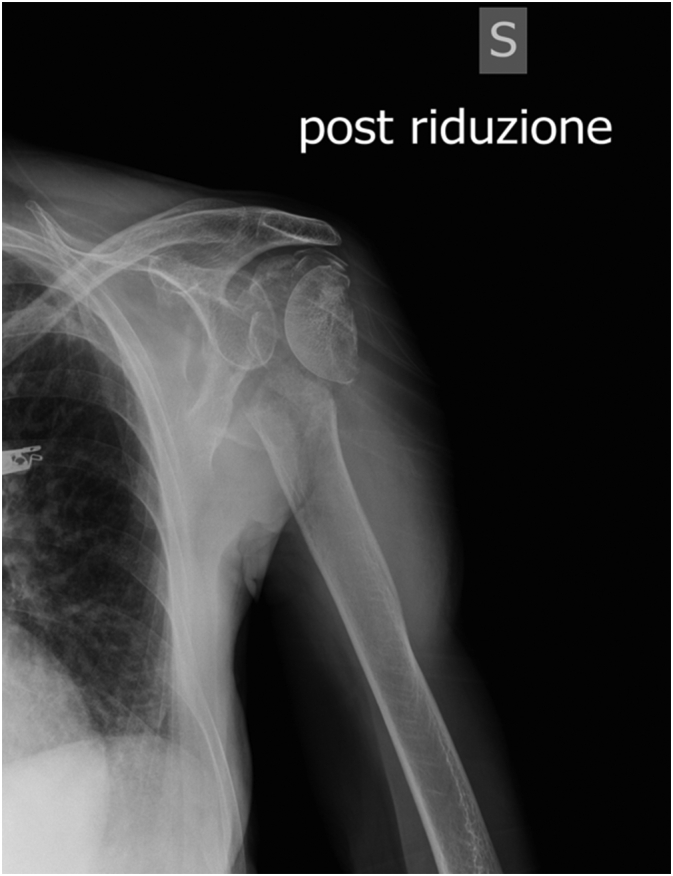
X-ray after attempt to reduction.
